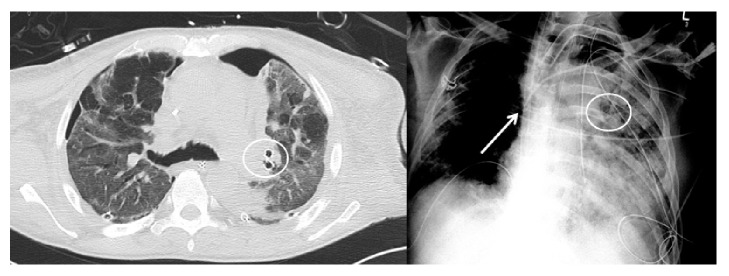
CT-scan (left image) and chest X-ray (right image) after endobronchial valve placement. The left lung is again fully expanded. The circled areas depict the two endobronchial valves within the left upper lobe segmental bronchi (LB1/2 and LB3). The Avalon Elite™ Bi-Caval Dual Lumen Catheter is visible on the chest X-ray (white arrow).
